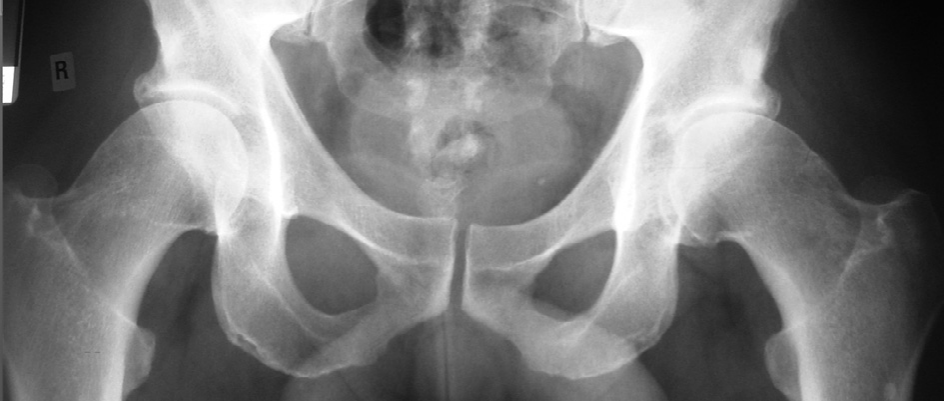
X-ray of the pelvis with both hips showing “pistol-grip” deformity of the proximal femur of both hips indicating “cam” type of impingement.
